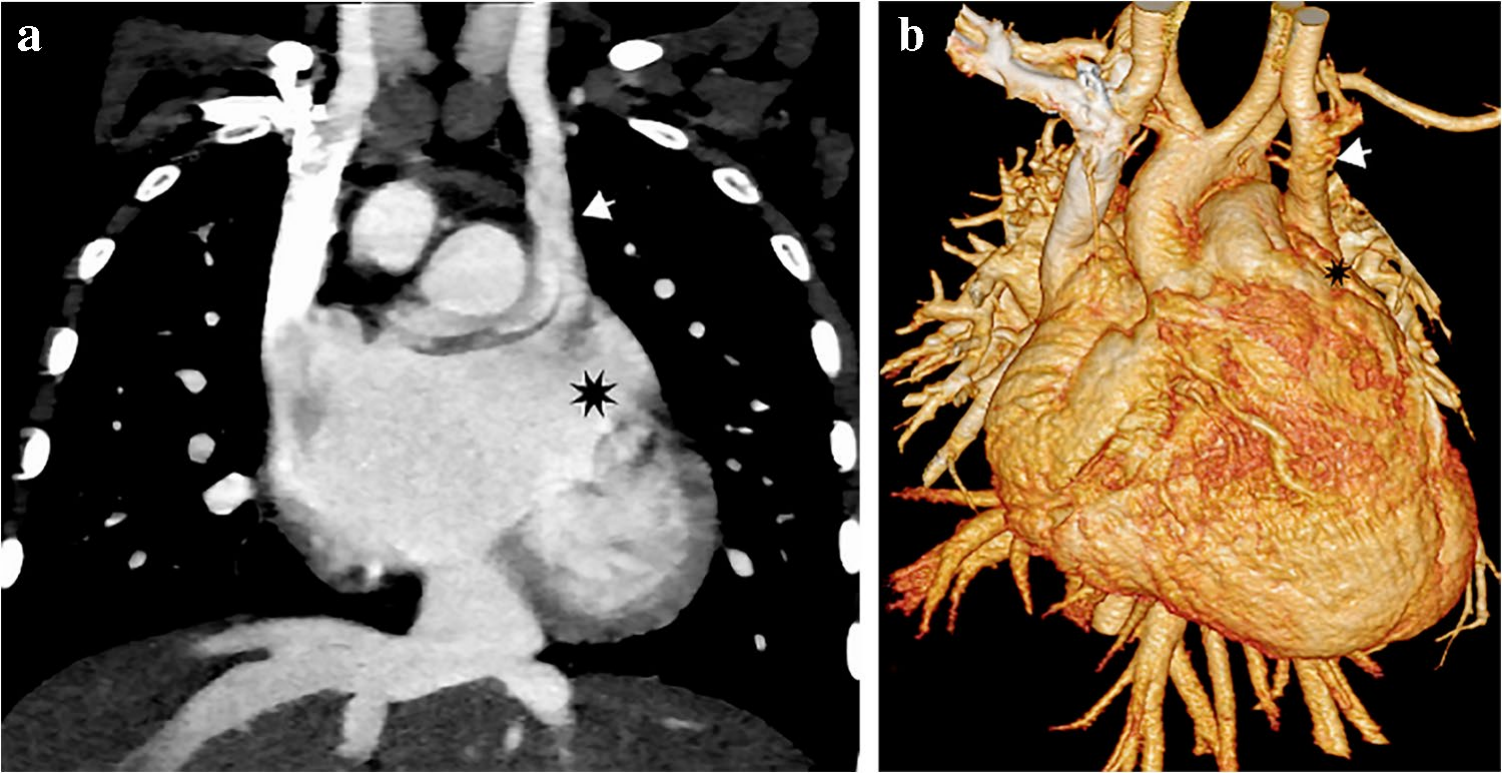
A persistent left superior vena cava (SVC) in a 2-year-old girl with left isomerism. a, b Coronal oblique computed tomography (CT) image (a) and volume-rendered CT image (b) of the persistent left SVC (arrows) show direct drainage into the left side of the common atrium (*asterisk*). The suprahepatic inferior vena cava drains into the posterior wall of the common atrium
